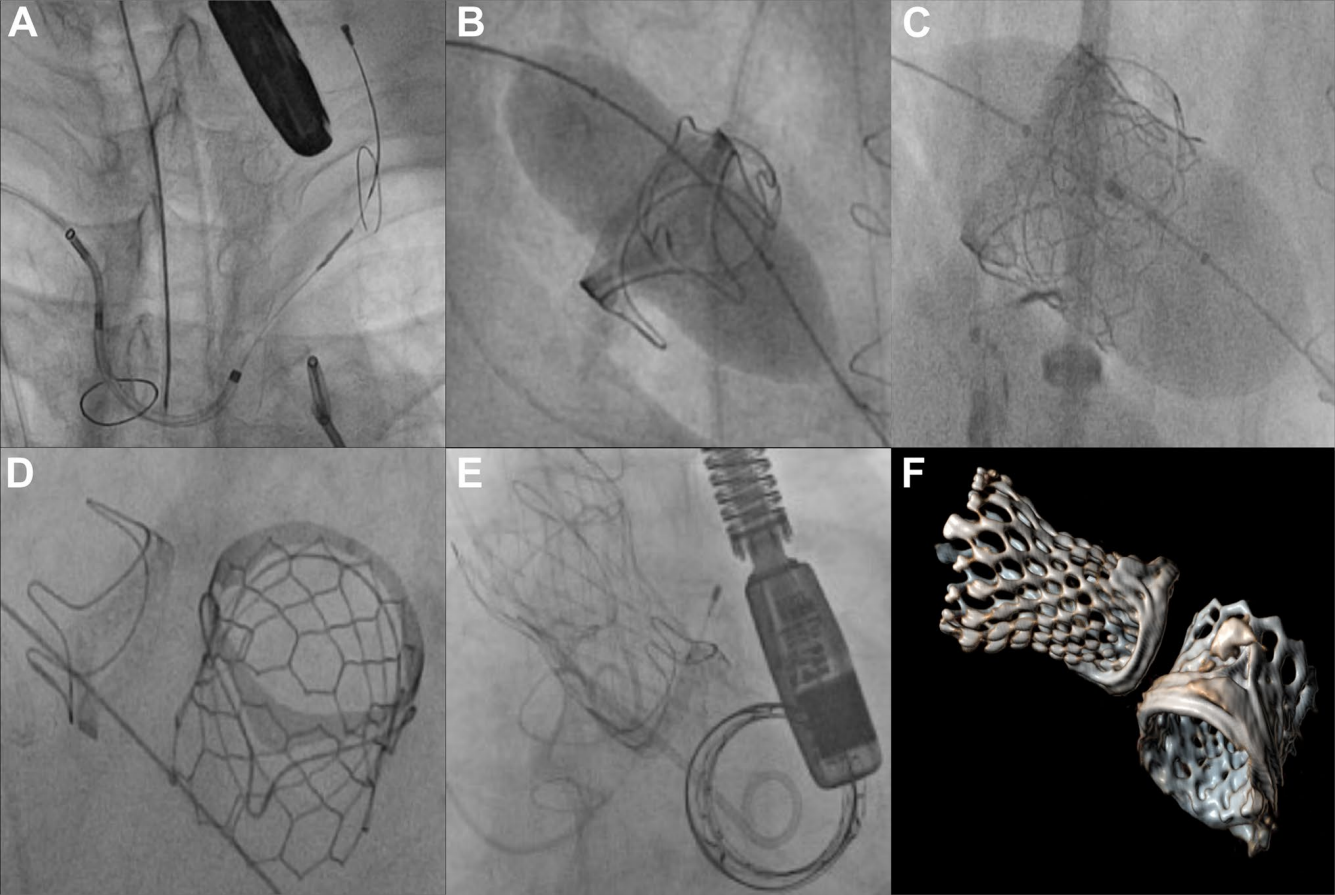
A Positioning of a Sentinel cerebral protection device in standard position covering brachiocephalic trunk and left common carotid artery. B Predilatation of the prosthetic mitral valve and C Implantation of the SAPIEN 3-Ultra 29 mm. D Result of ViV replacement in mitral position. E Result of ViV replacement in aortic position after implantation of Evolut R 26 mm. F Follow-up CT revealed well-seated transcatheter heart valves in aortic and mitral valve position
